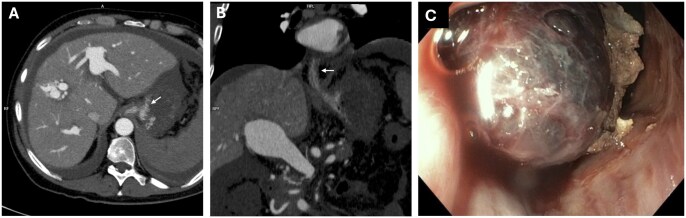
CT scan portal phase showing gastric (A, axial) and oesophageal varices (B, coronal). Upper endoscopy demonstrating a large bleeding gastro-oesophageal varices type 1 treated with cyanoacrylate injection (C).
